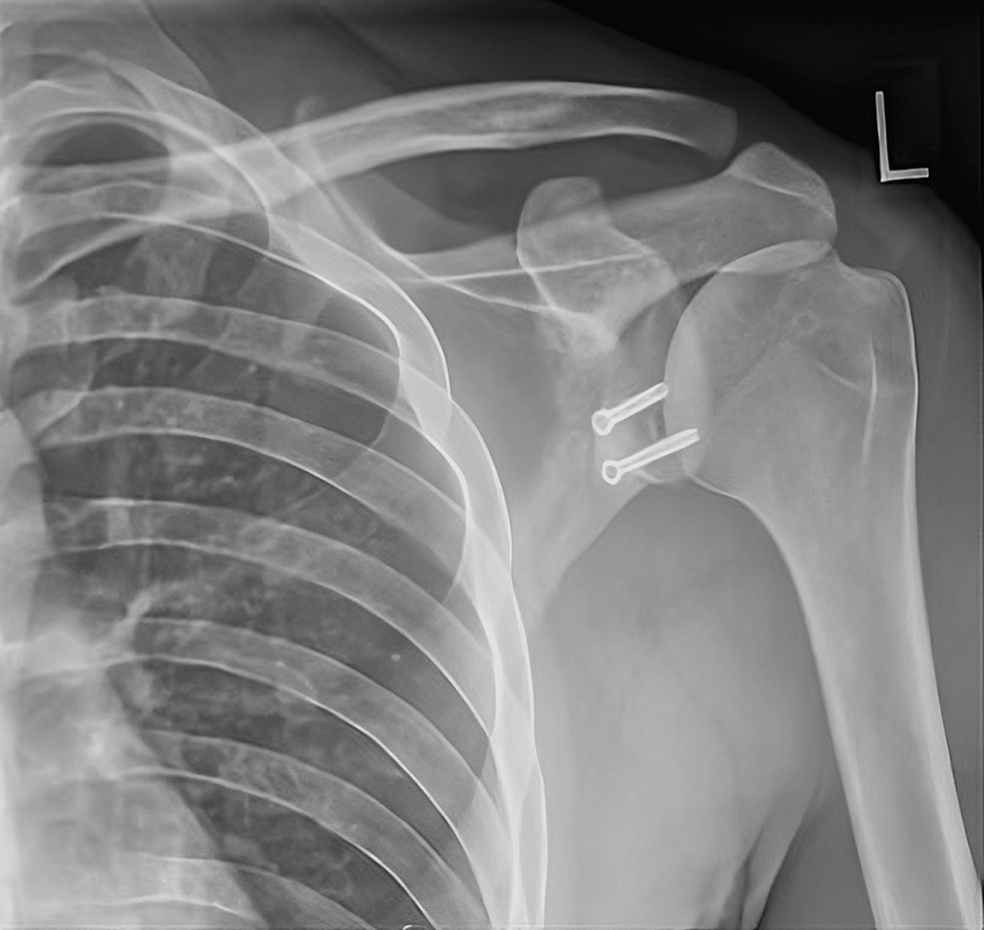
Radiograph (anterioposterior view) showing postoperative left shoulder Latarjet procedure.
